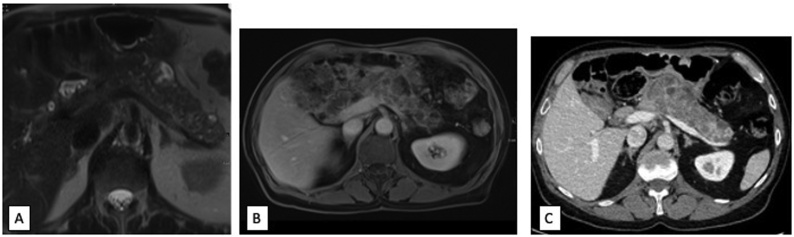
(A) Axial T2 weighted MR abdomen showing enlargement of the pancreatic body and tail with multiple new cystic lesions 8 months post-operative from the index operation. (B) Axial post-contrast MR abdomen at 11 months post-operative from the index operation and (C) axial contrast enhanced CT abdomen showing heterogeneous contrast enhancement of the body and tail at 15 months post-operative from the index operation.
